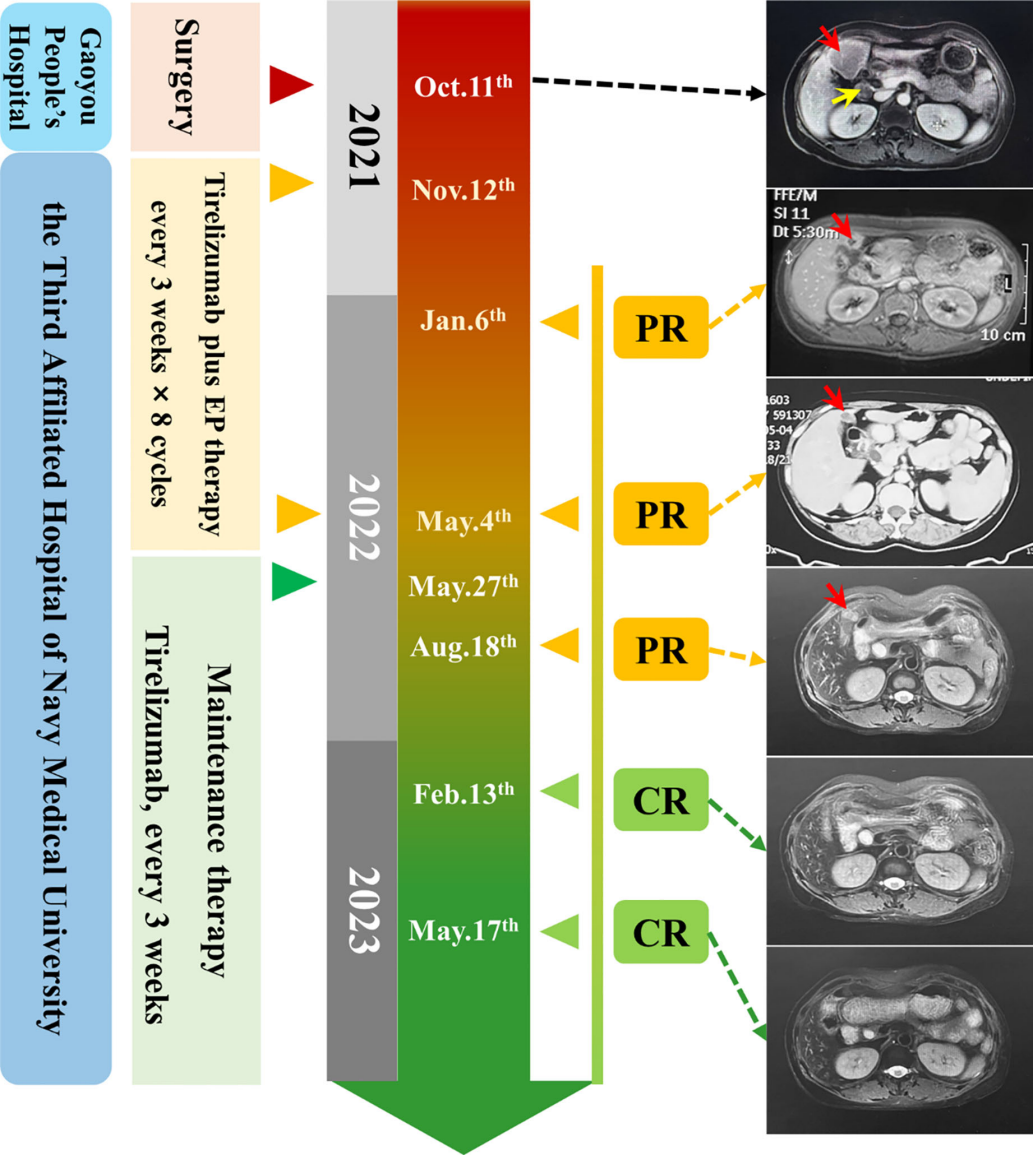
Timeline of the clinical course. Right: MRI and CT images of baseline (12 November, 2021) and after therapy (red array: metastatic cancer in the liver, yellow array: metastatic lymphoma nodes).
